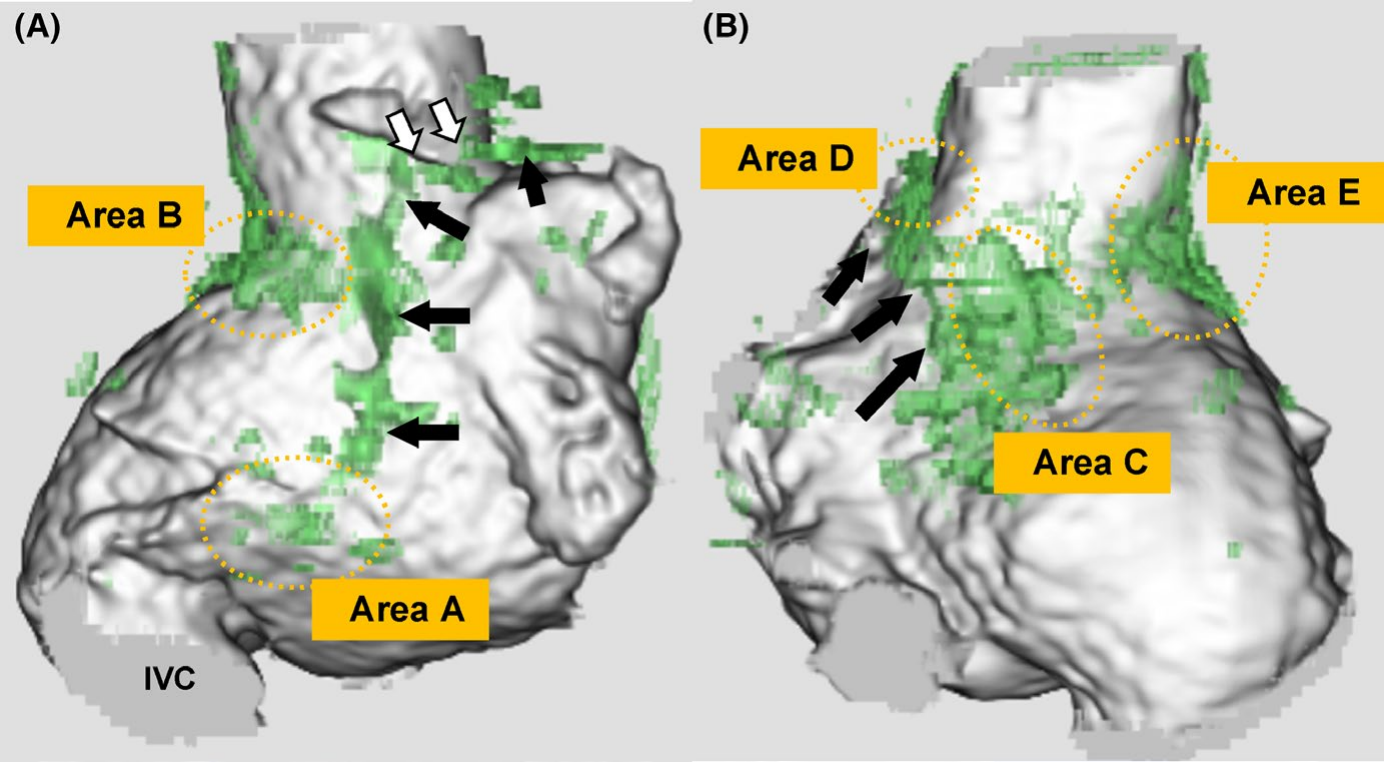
3D LGE‐MRI. 3D LGE‐MRI of the RA in the right anterior oblique (RAO) (A) and posterior‐anterior (PA) (B) views. The green areas indicate LGE. The black arrows show the long linear LGE, and the yellow dotted circles show the patchy one. The white arrows indicate the visual gap of the LGE. LGE‐MRI, late‐gadolinium enhancement magnetic resonance imaging; RA, right atrial; RAO, right anterior oblique; PA, posterior‐anterior
